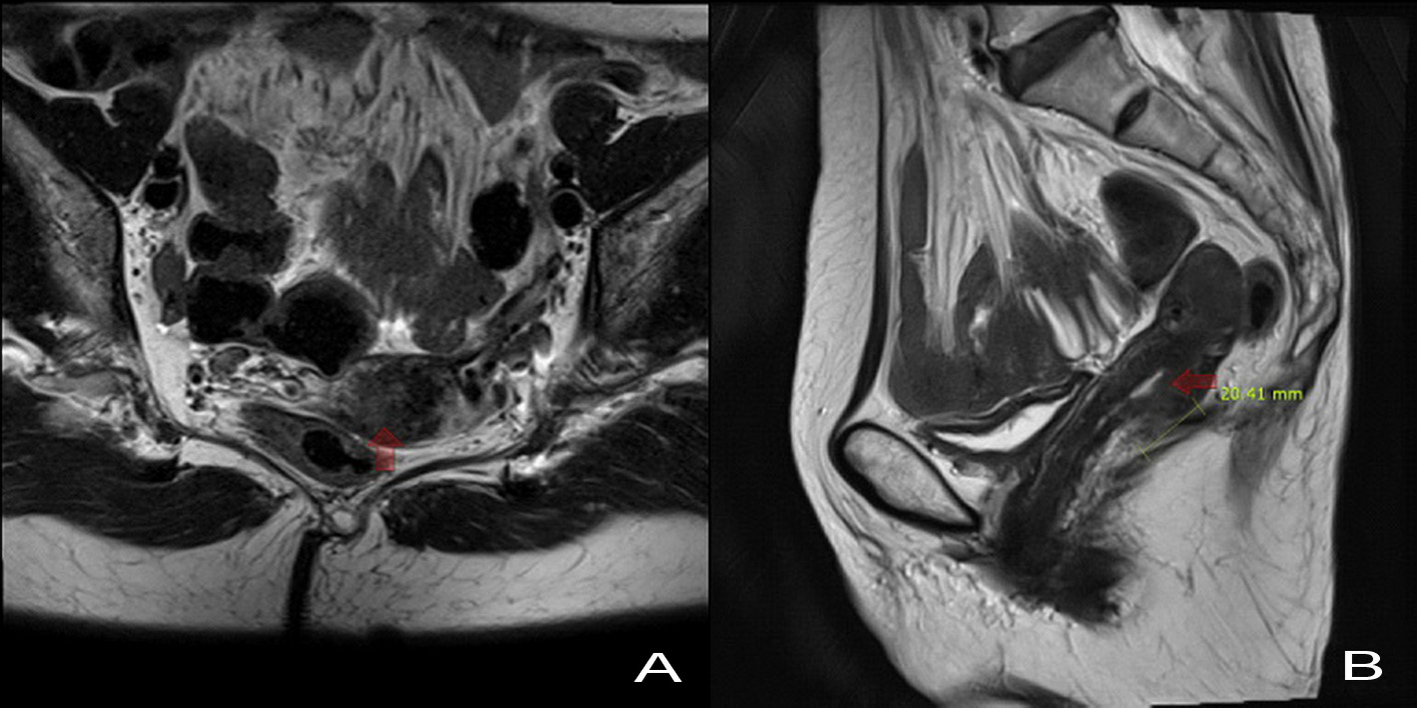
T2-weighted pelvic magnetic resonance images on the axial plane (A) and sagittal plane (B). T2-weighted pelvic magnetic resonance images on the axial plane (A) and sagittal plane (B). Note the cervical mass-like lesion (infiltrative wall thickening with signal enhancement, 2.0 × 0.6 cm) at posterior portion of the uterine cervix with likely posterior vaginal fornix involvement. (arrows point to cervical lesion)
